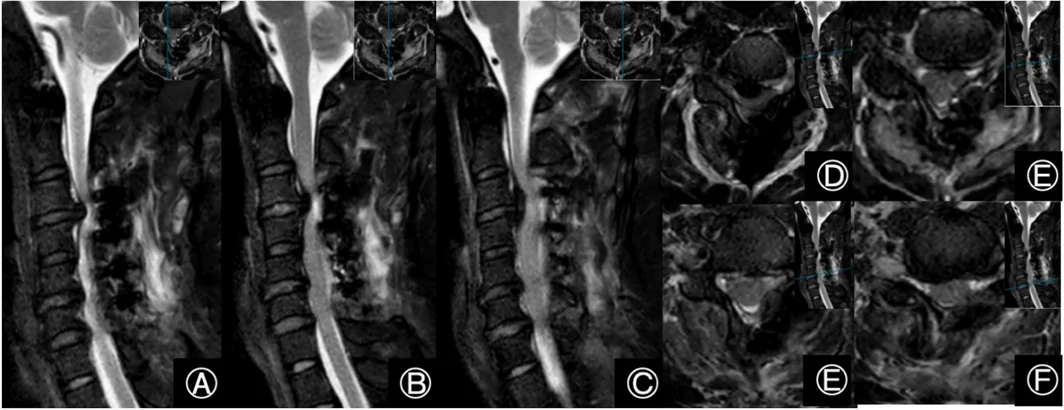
Cervical MRI on postoperative Day 3. The follow‐up imaging displayed that the spinal cord compression was partially relieved, with inadequate posterior migration observed at C3 and C4 levels.
